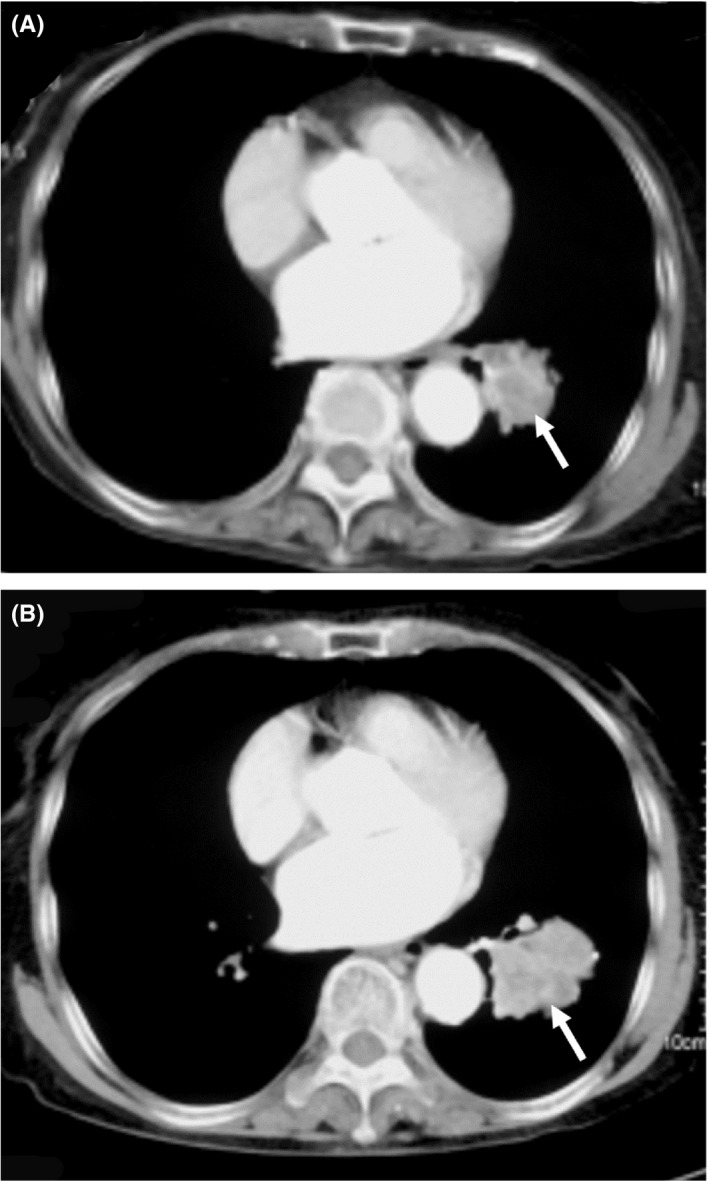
(A) The initial contrast‐enhanced axial plane CT thorax in the soft tissue window in September 2019 showed a tumor mass (white arrow). (B) The size of the tumor mass (white arrow) was reduced 16 months later, compared with the initial scan.
